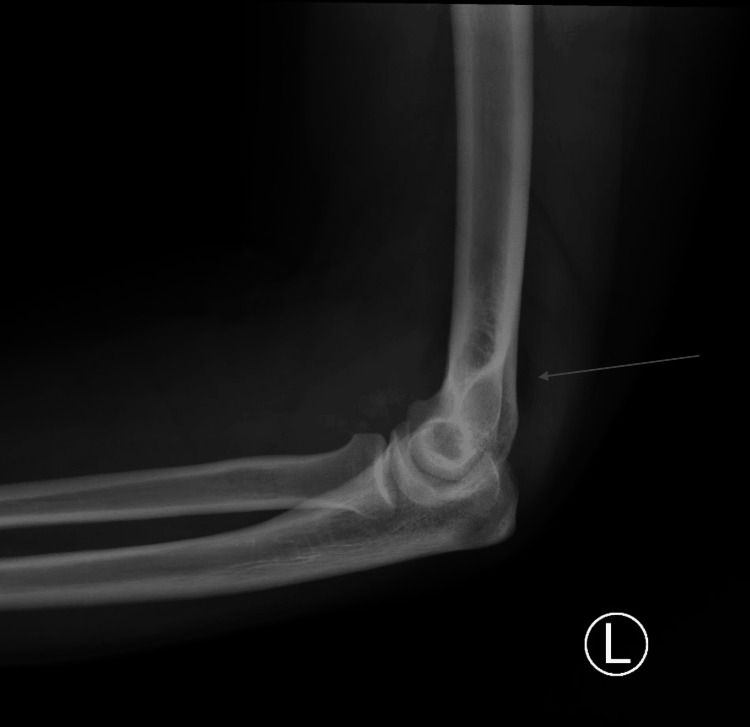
X-ray of the left elbow with posterior fat pad. The arrow indicates the posterior fat pad consistent with elbow effusion.
